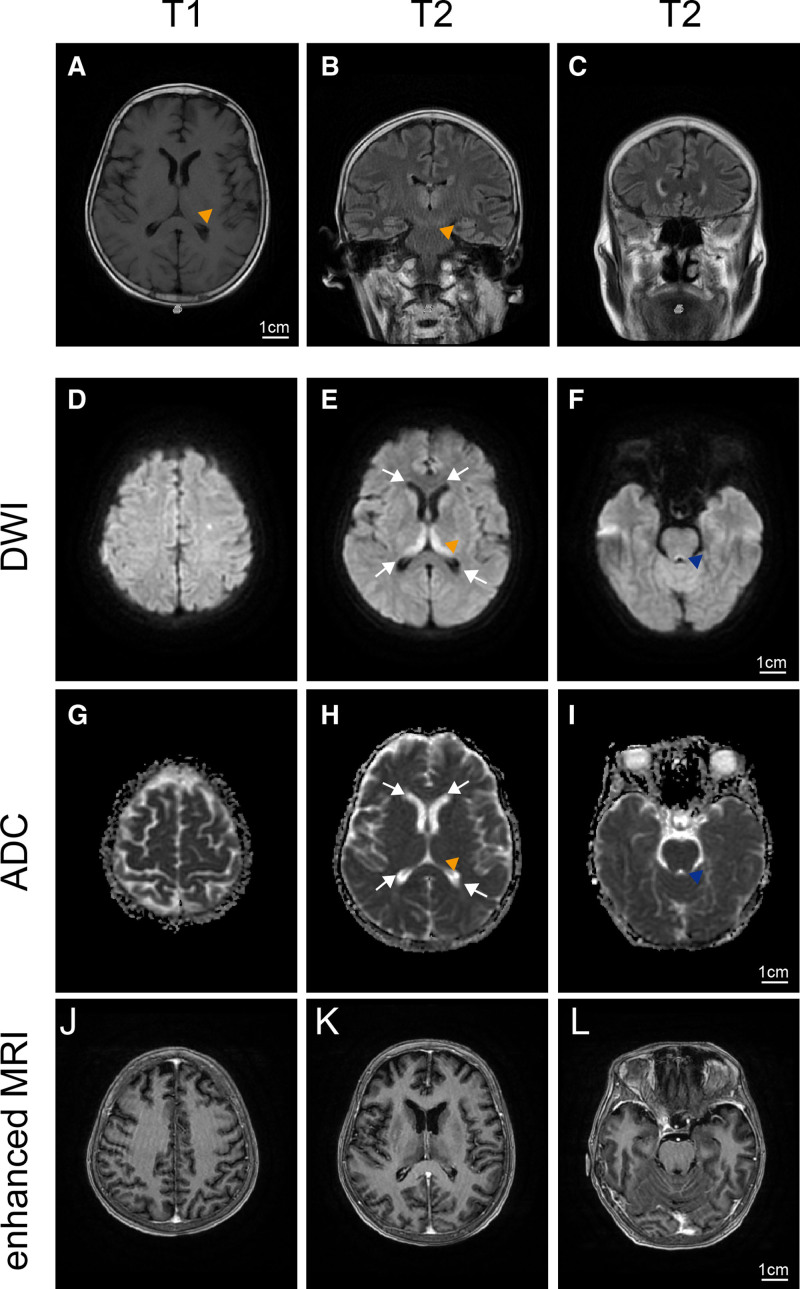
(A to L) MRI images acquired on the 9th day post admission. (A) T1 showed slightly low signal intensity in the internal bilateral thalamus. (B and C) T2 FLAIR showed high signal intensity in the internal bilateral thalamus (yellow triangle), bilateral regions adjacent to the lateral ventricles. (D) Diffusion-weighted imaging (DWI) did not demonstrate abnormal signals in bilateral frontal and parietal lobe. (E and F) DWI revealed high signal intensity in the internal bilateral thalamus (yellow triangle), bilateral caudate nucleus head (white arrows), and the periaqueductal area (blue triangle) at the posterior margin of the brainstem. (G) Apparent diffusion coefficient (ADC) did not demonstrate abnormal signals in bilateral frontal and parietal lobe. (H and I) ADC revealed slightly low signal intensity in the internal bilateral thalamus (yellow triangle), bilateral caudate nucleus head (white arrows), and the periaqueductal area (blue triangle) at the posterior margin of the brainstem. (J, K, and L) Enhanced MRI in the same regions did not show abnormal enhancement. Scale bar = 1 cm. MRI = magnetic resonance imaging.
